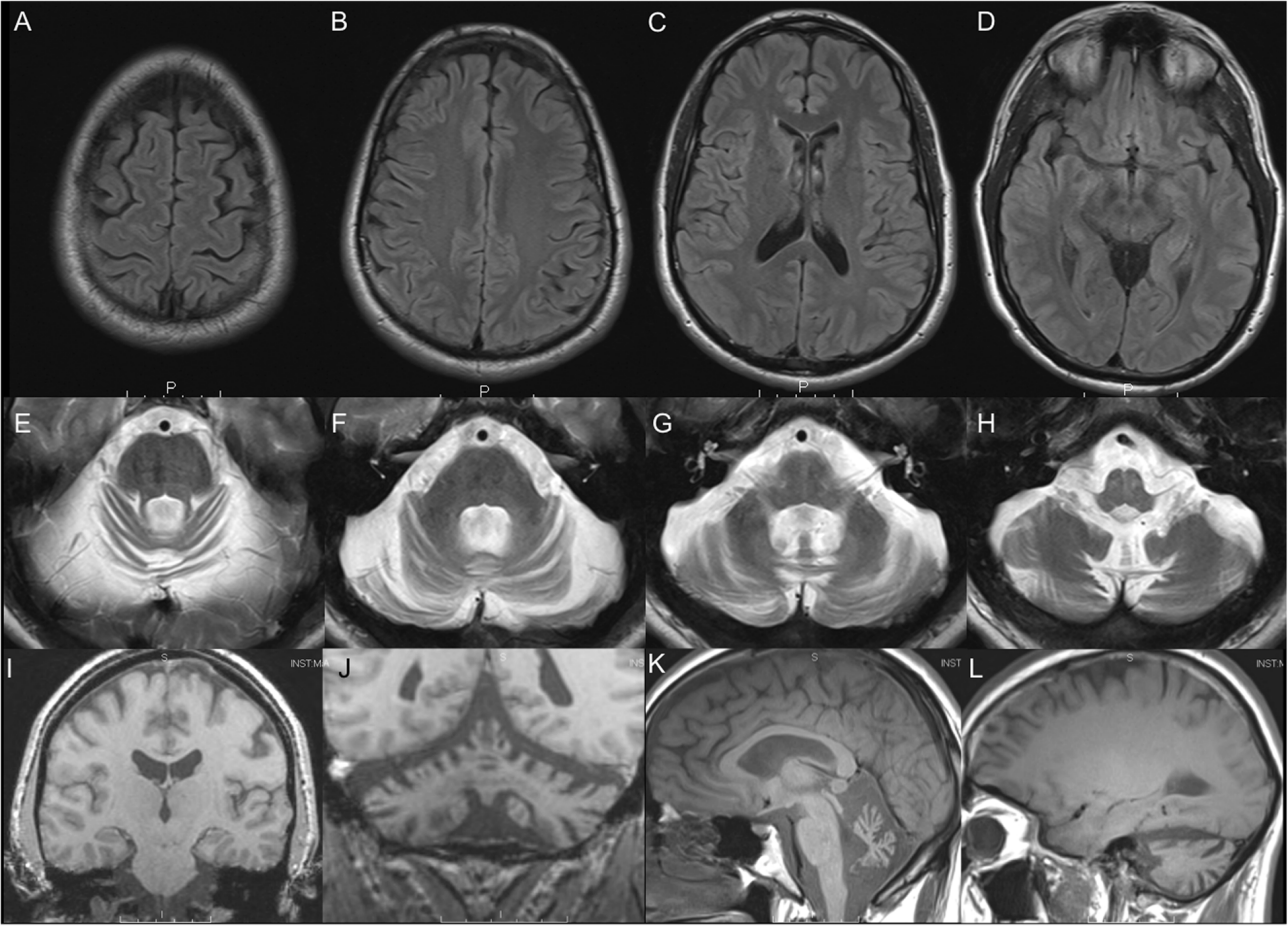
MRI at age 35 demonstrating cerebellar volume loss with preservation of cerebral hemispheres. A-D) FLAIR axial; E-H) T2 axial; I-J) Coronal T1; K-L) Sagittal T1.
